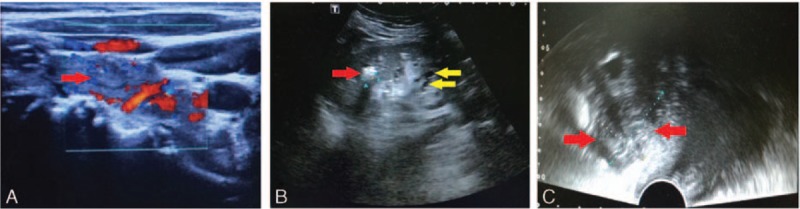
Color ultrasonography examination. (A) Thyroid color ultrasonography showed multiple nodules with high echo (a 21 mm × 11 mm high echo thyroid nodule was represented by a red arrow). (B) Right kidney stone (a single hyperechoic nodule of 11 mm × 8 mm with acoustic shadow, represented by a red arrow), right kidney cysts (represented by yellow arrow); (C) hysteromyoma (red arrow).
